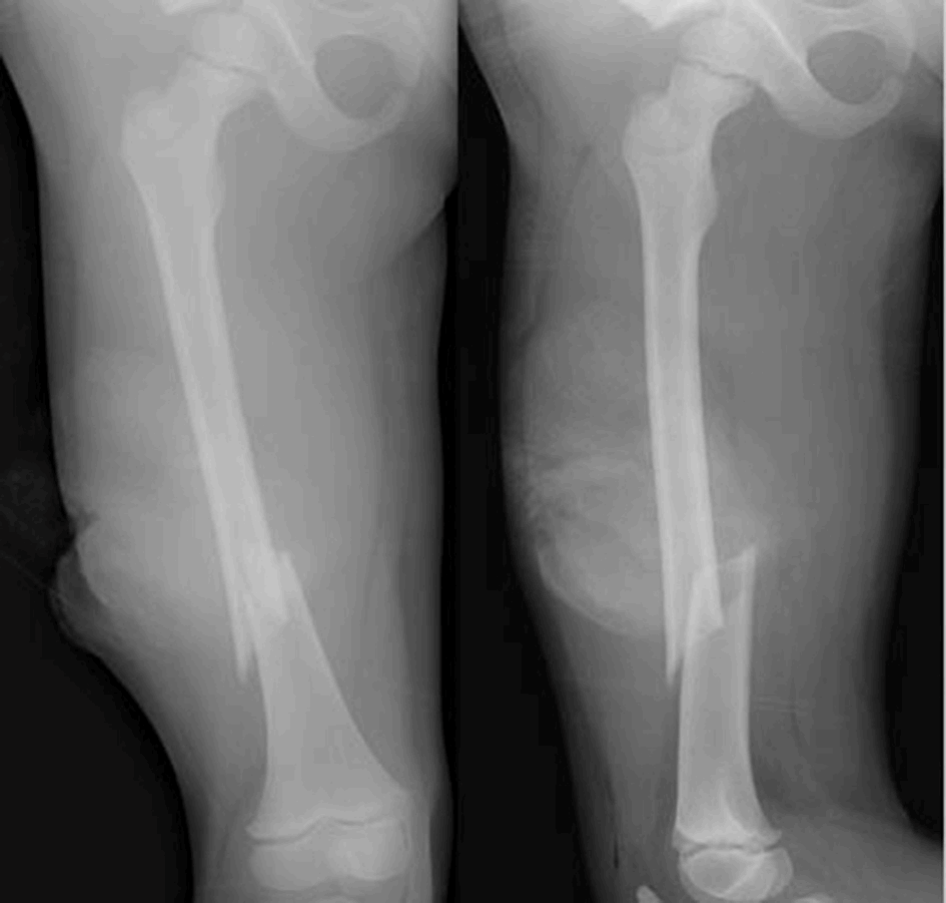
X-ray showing right femur fracture.
